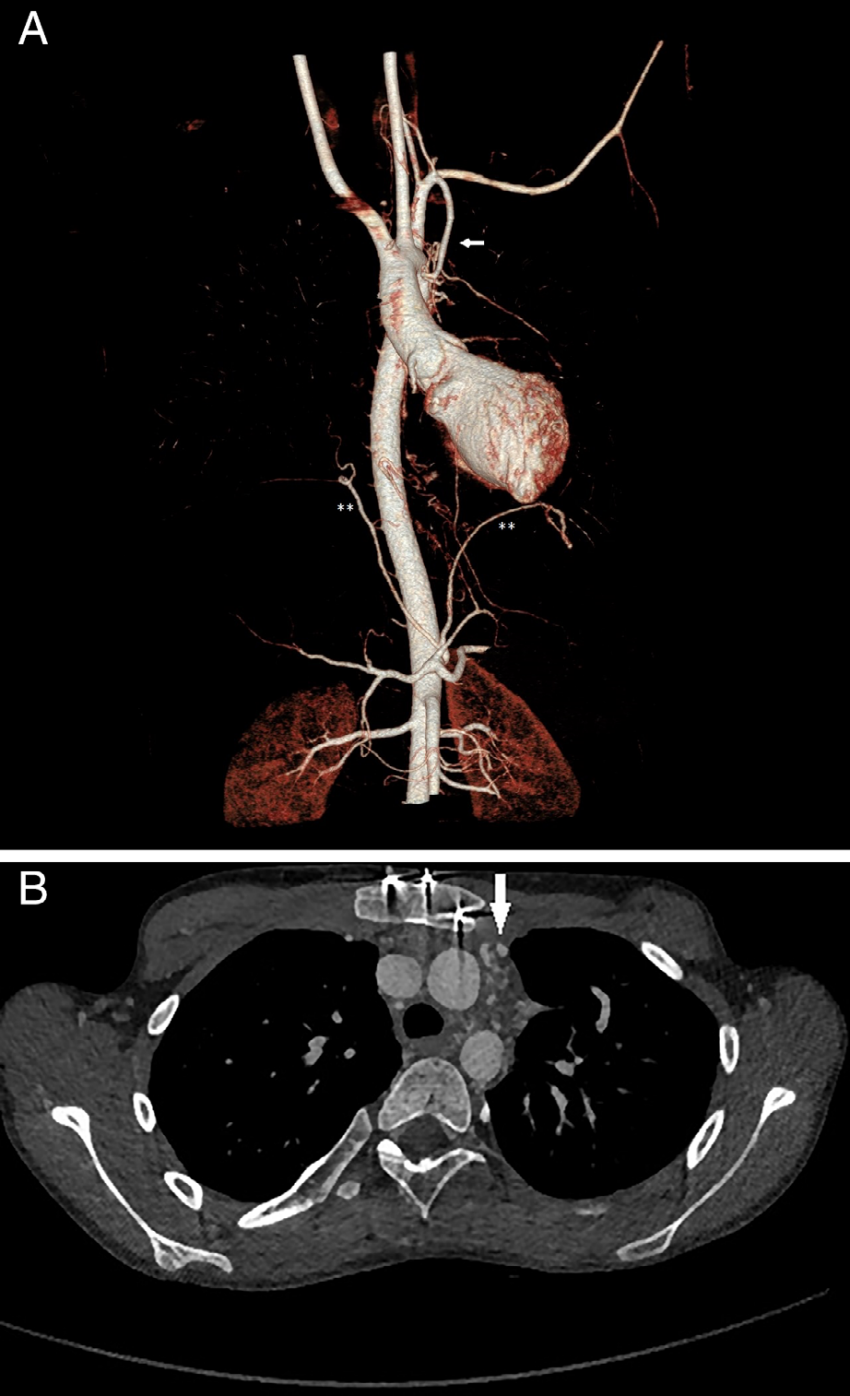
Computed tomography (CT) angiogram of the chest with (A) volume rendering technique and (B) axial images demonstrating hypertrophied left internal mammary (arrow) and bilateral inferior phrenic arteries () supplying collaterals to bronchi in the superior mediastinum. No ground‐glass changes were noted to suggest pulmonary haemorrhage.
